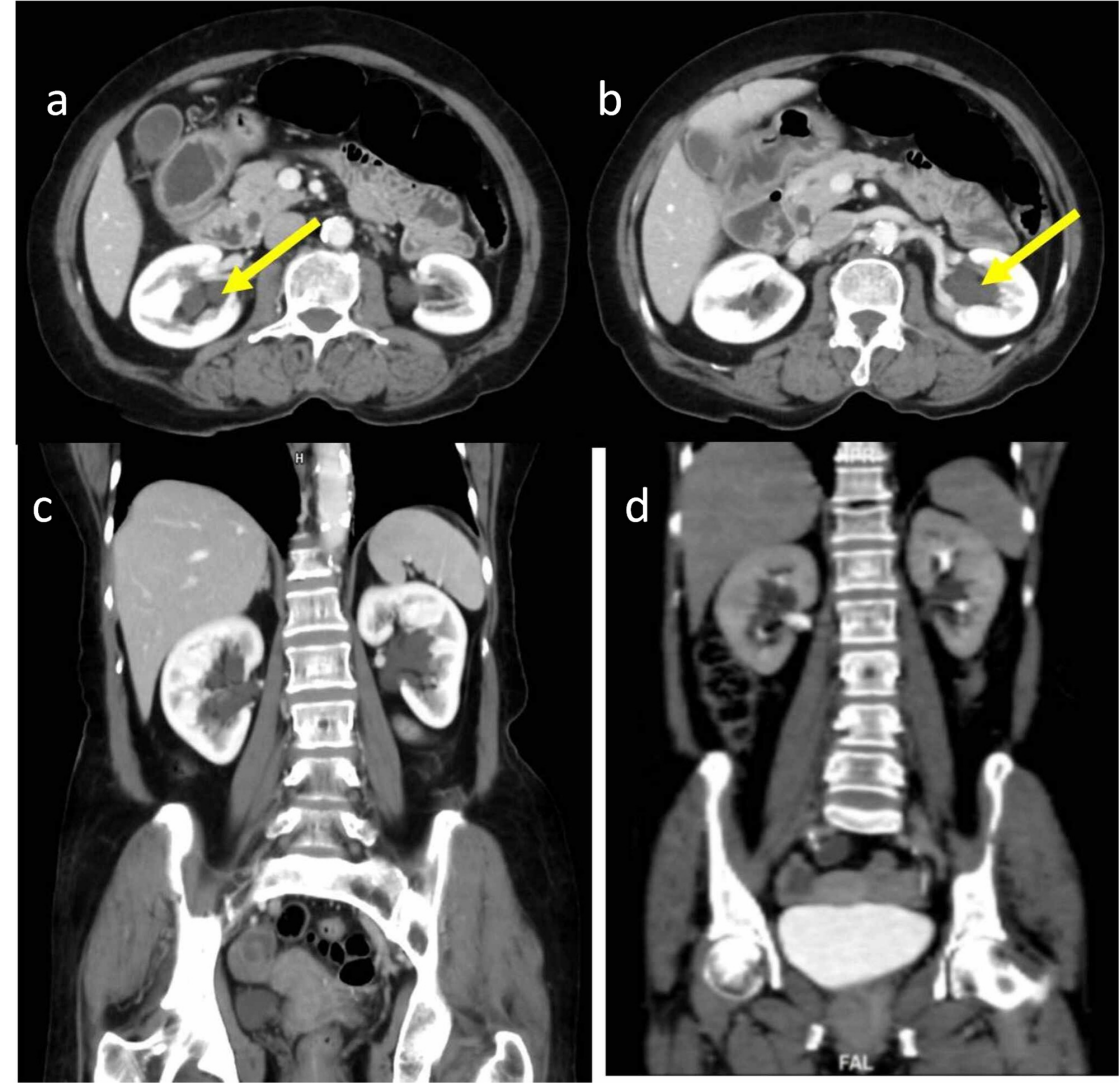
69-year-old female with bilateral peripelvic renal lymphangiectasia Contrast-enhanced CT of the abdomen in axial (a, b) and coronal (c) planes in the venous phase demonstrates lobulated low-density peripelvic lesions (yellow arrows) in both kidneys splaying the collecting system. Coronal contrast-enhanced CT of the abdomen in the delayed phase in the coronal plane (d) demonstrates no opacification of contrast in the low-density peripelvic lesions CT: computed tomography
